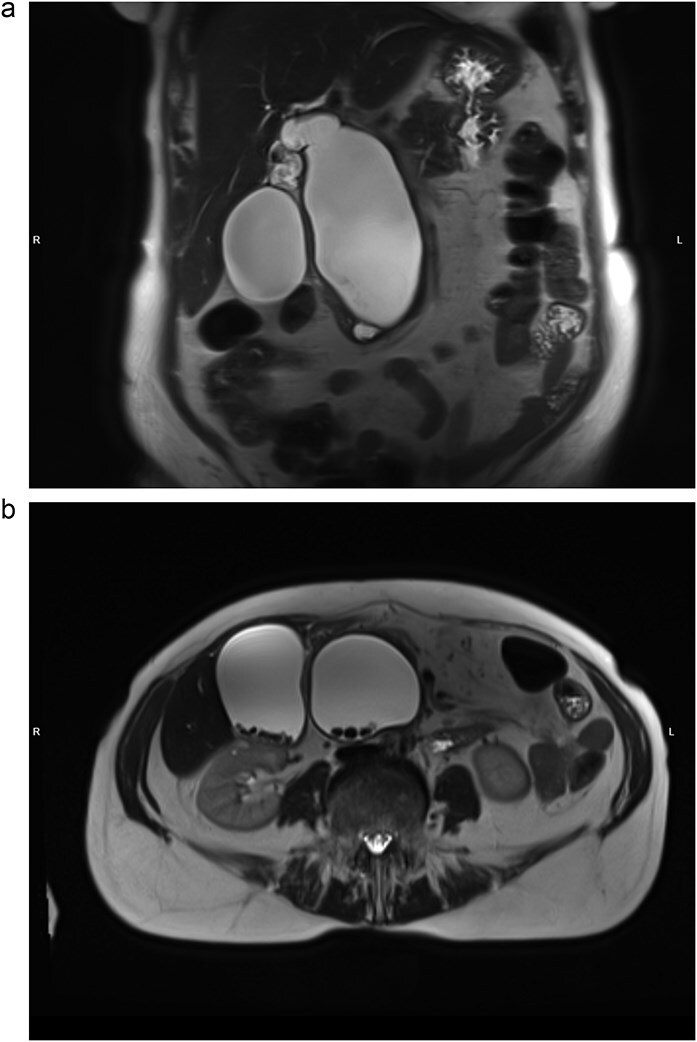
MRI, T2-weighted scan. Todani type IA biliary cyst. (a) Coronal slice: cystic dilation of CBD, diffusely dilated biliary tree; (b) axial slice: multiple CBD and gallbladder stones.
